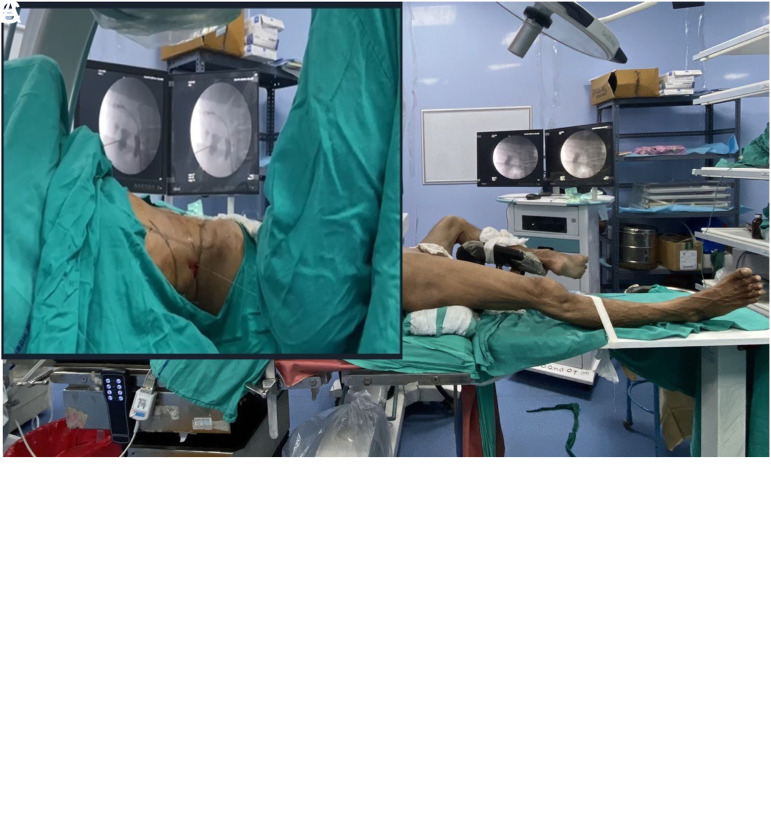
(A) Calcutta position in patient with kyphoscoliosis with right duplex system. (B) X-ray showing kyphoscoliosis with right renal calculus. (C) Initial puncture done using triangulation technique in middle calyx.
